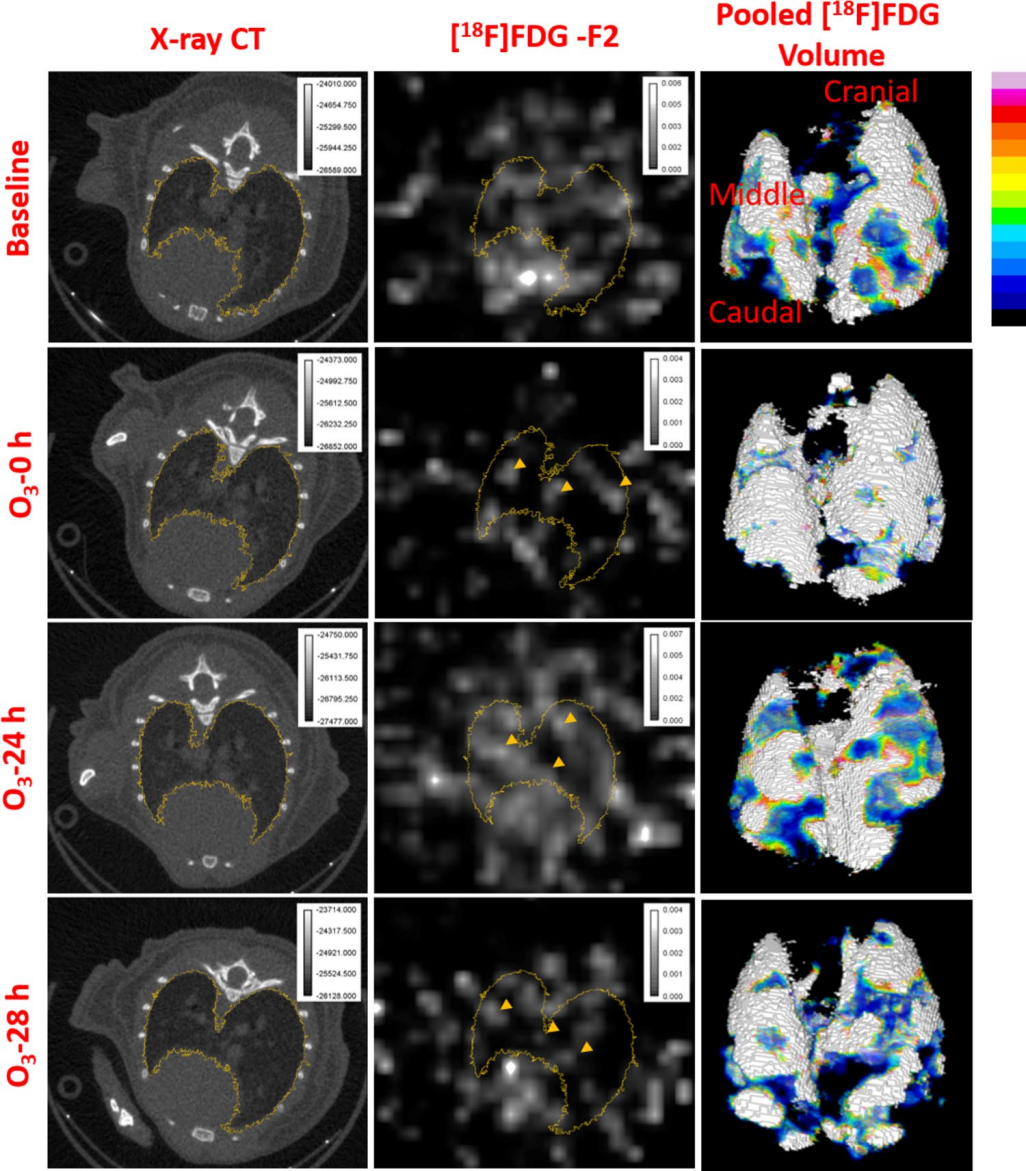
Sequential lung [^18^F]F-FDG distribution using X-ray region-of-interest: representative lung X-ray CT slice, FDG slice in the third frame (F2) and a 3-d render of the merged lung [^18^F]F-FDG volume at baseline, O_3_-0, 24 and 28 h. The 3-d render shows [^18^F]F-FDG activity normalized to16-color scale. The yellow arrow-heads indicate regions of high lung [^18^F]F-FDG uptake.
